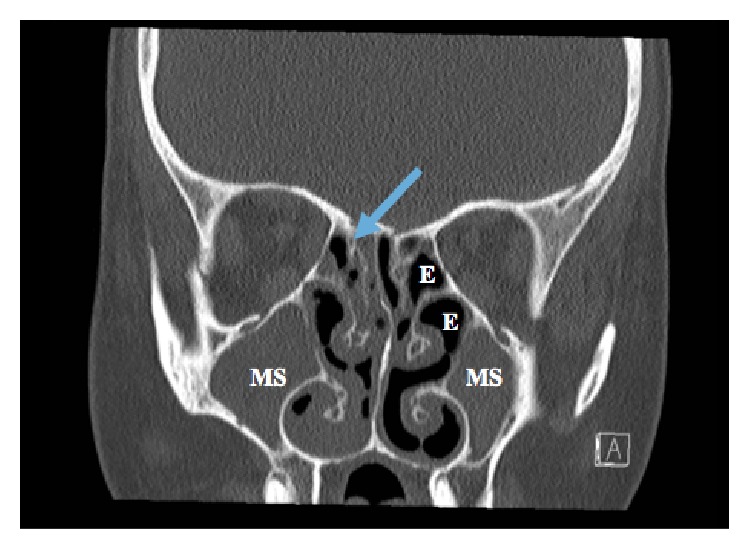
CT scan of the paranasal sinuses showing complete opacification of the maxillary sinuses (MS) bilaterally and some of the ethmoid air cells (arrow) including the sphenoid sinuses. There is also obliteration of the osteomeatal complexes bilaterally.
